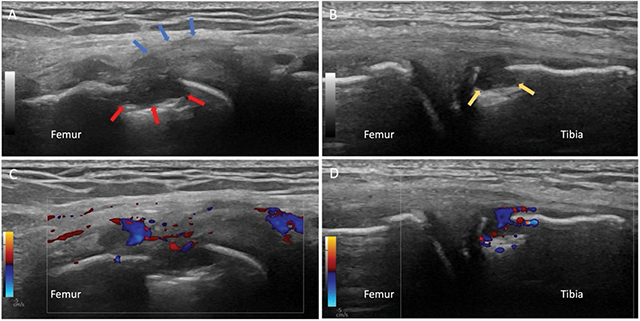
Ultrasound of the right knee demonstrating a thickened popliteal tendon and erosion of the the popliteal sulcus (A and C). A second erosion was shown on the medial rim of the medial tibial plateau (B and D).
